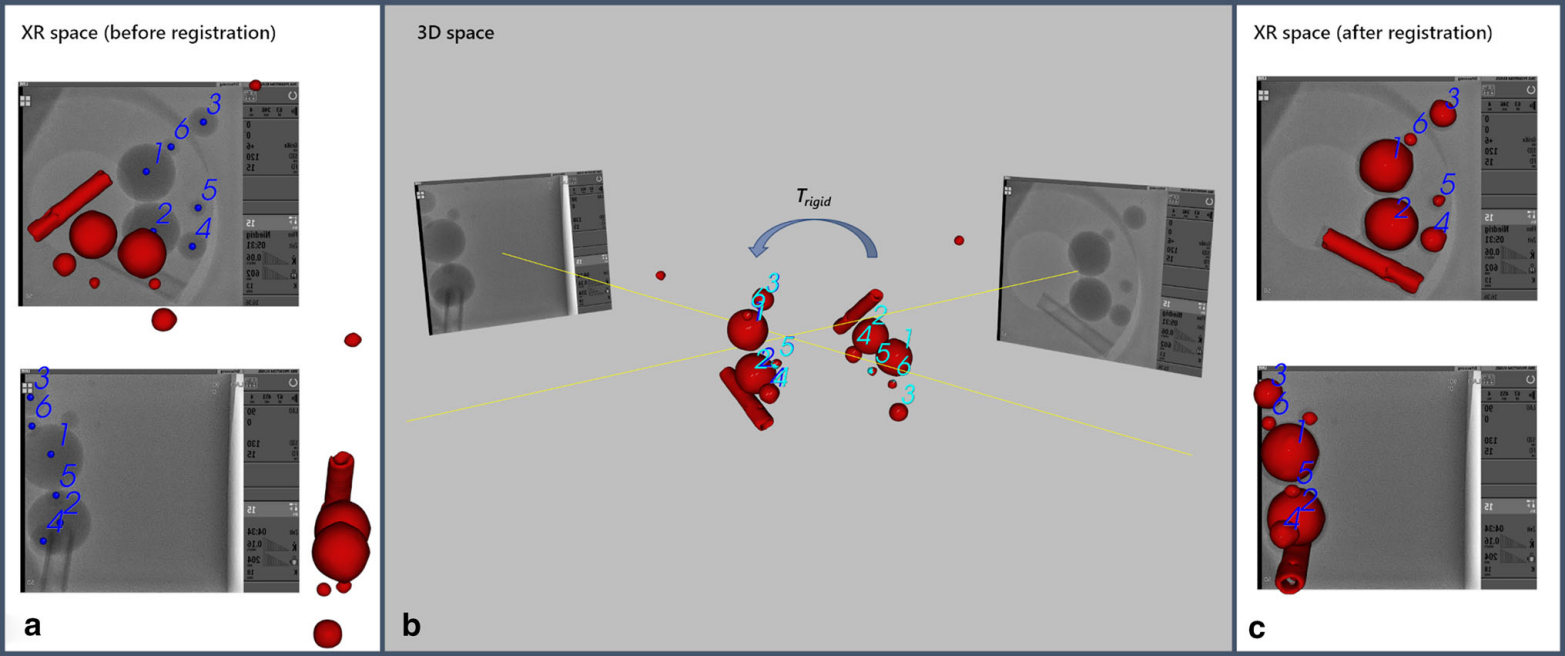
Registration between 3D MRI and 2D XR space: a two sets of six corresponding 2D points are manually identified in the center of respective glass spheres (blue) in two orthogonal XR views; b 3D scene with 3D points reconstructed from corresponding 2D points identified in each XR view (blue) and corresponding 3D MRI points (turquoise) before T_rigid_ registration (sets of blue and turquoise points are spread in space) and after (sets of blue and turquoise points are aligned); c result of the registration in XR space as 2D overlays in two orthogonal views
